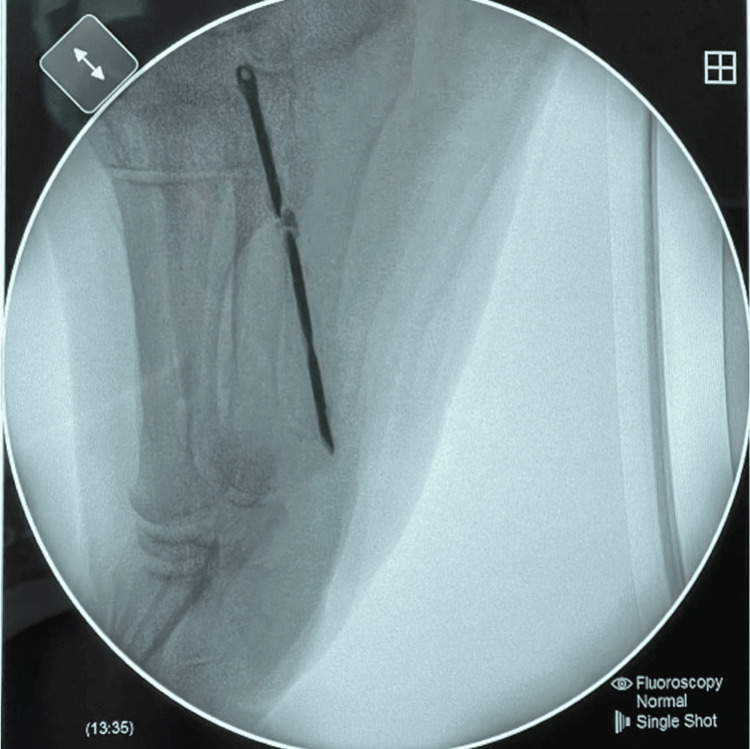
X-ray taken during the operation.
